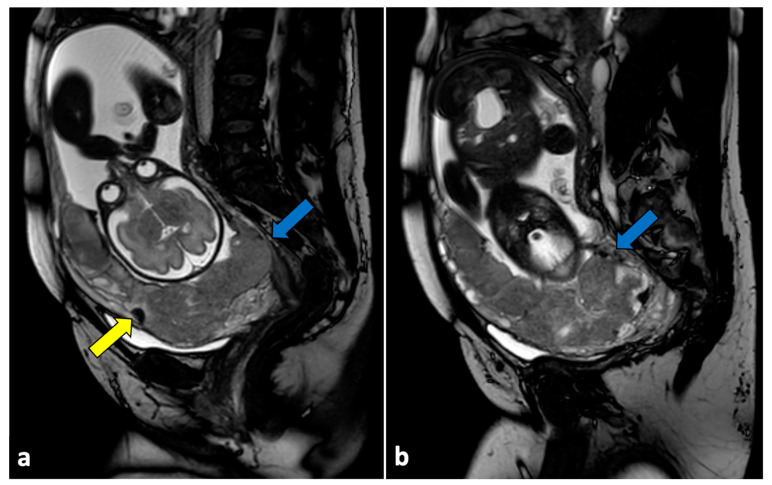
Placenta accreta in a 33-year-old patient at 36 weeks of gestation. Sagittal (a,b) T2-weighted MR images show a placenta previa (blue arrows) covering the uterine internal os. Placenta has irregular contours and rounded edges, with a single area of intraplacental dark T2 bands (yellow arrow). No interruptions in the thin hypointense myometrial border are seen. Pathologic examination confirmed the diagnosis of placenta accreta.
